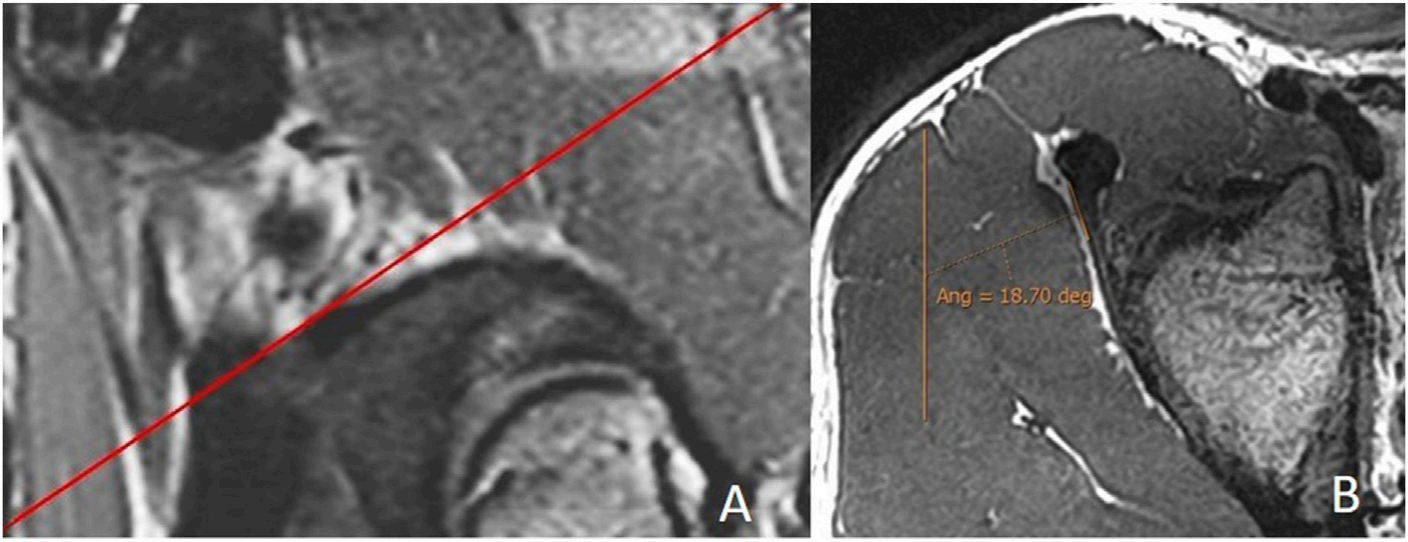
Measurement of the indirect tendon slope. (A) Sagittal 3D proton density with the reference line to obtain the batch of the oblique axial reconstruction following the orientation of the indirect tendon. (B) Median section of the oblique axial reconstruction of the indirect tendon with measurement of its inclination (indirect tendon anterolateral margin/anteroposterior axis). The portion of the CT is excluded. In this case, the merging of the indirect tendon with the CT in the axial plane is more lateral than the acetabular insertion of the indirect tendon, and the inclination is negative (−18.7°).
